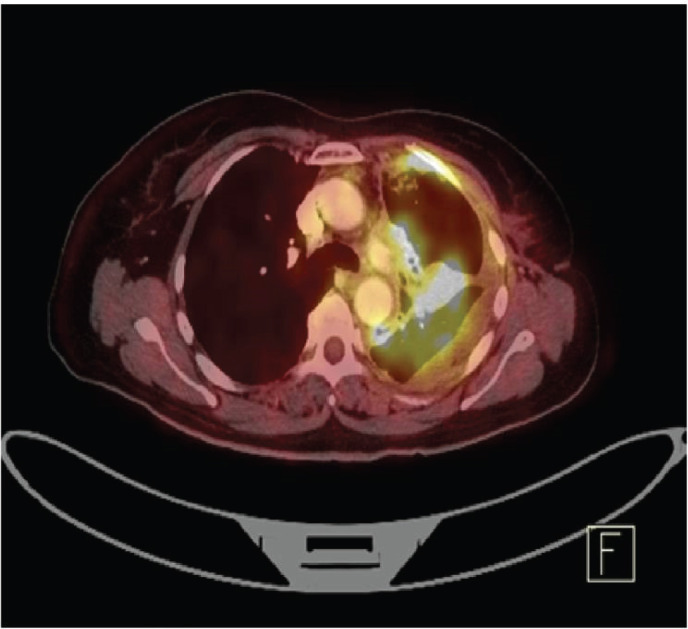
PET-CT showing metabolically active metastatic lesion in the upper lobe of left lung along with metabolically active moderate left side pleural effusion.
